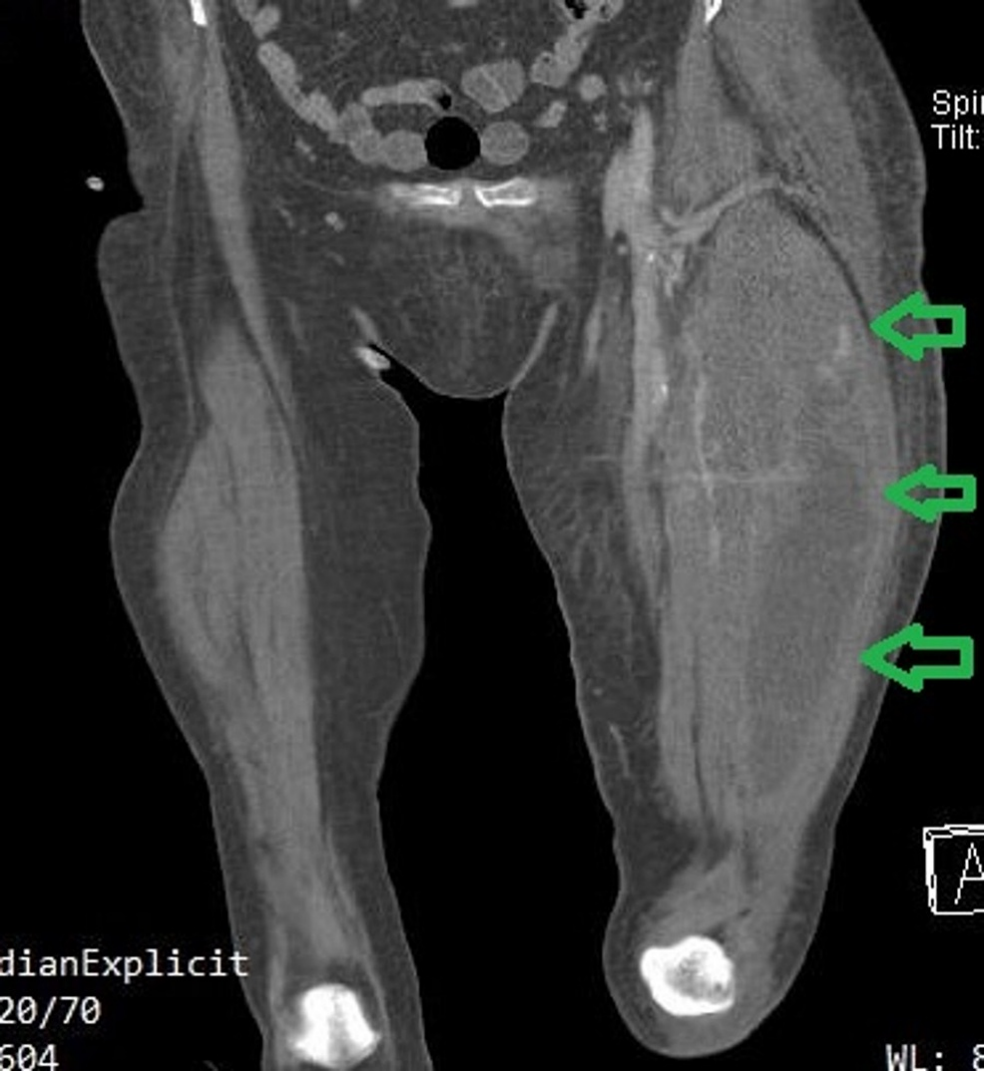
Coronal computed tomography angiography image indicates the huge hematoma formation of the left thigh (green arrows).
